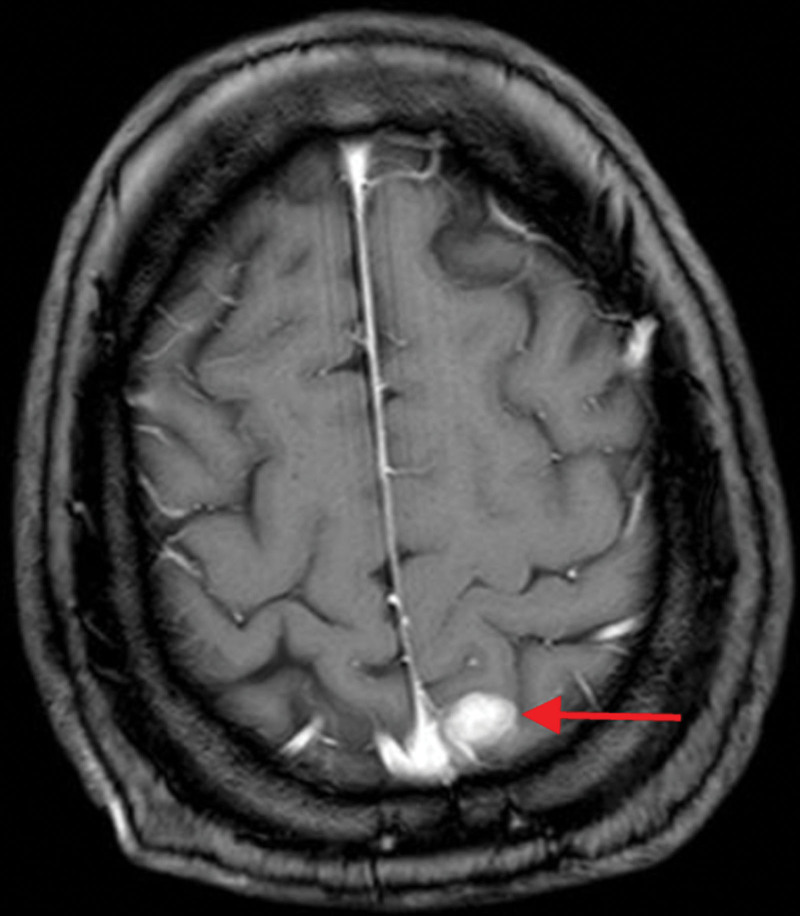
Enhanced MRI of the head showing the presence of an enhanced nodule (arrow) adjacent to the falx cerebri in the left parietal lobe (before SRS). MRI = magnetic resonance imaging, SRS = stereotactic radiosurgery.
